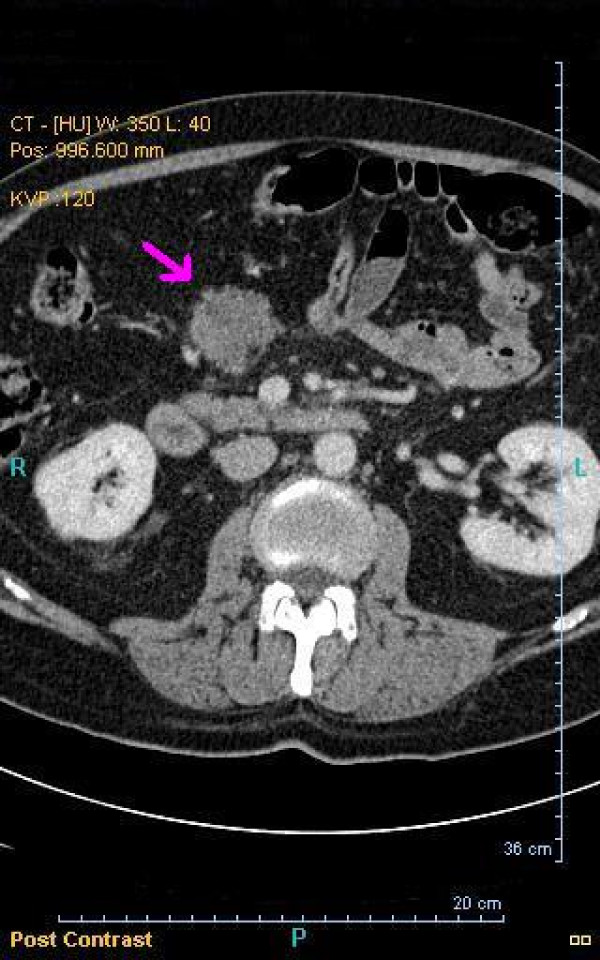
A section of computerized tomography [CT] scan. Arrow identifies the infrapyloric mesenteric mass.
